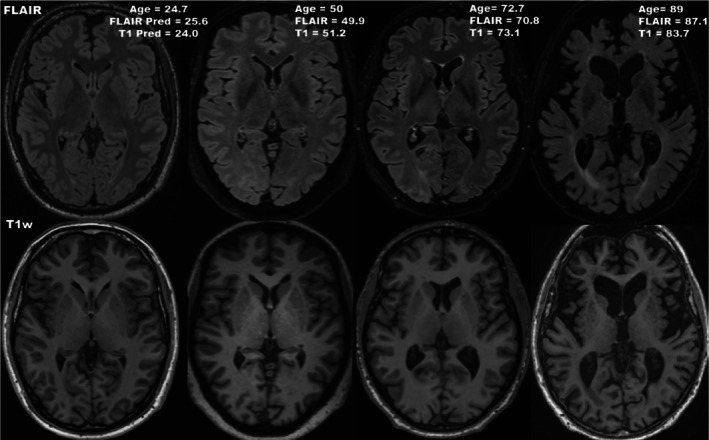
T2‐FLAIR (top) and T1w (bottom) MRIs of healthy controls of varying ages. With increasing age, the size of the ventricles can be seen to enlarge, and the sulci widen. At older ages, white matter lesions and thinning of the gray matter can be seen. Gray matter thinning can be better appreciated on T1w images, while white matter lesions are better seen on T2‐FLAIR scans. Brain‐predicted ages (Pred) are shown from our final FLAIR and T1w models combined (ensemble outputs averaged). Subjects were taken from the test set (unseen during training).
